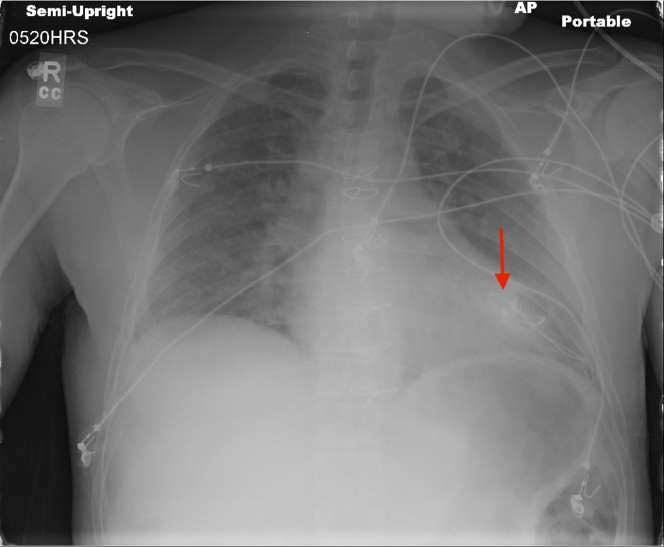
Chest X-Ray Demonstrating Retained Epicardial Pacing Leads (Arrow) Attached to the Heart and Adjacent to the Pericardium After Generator Removal
